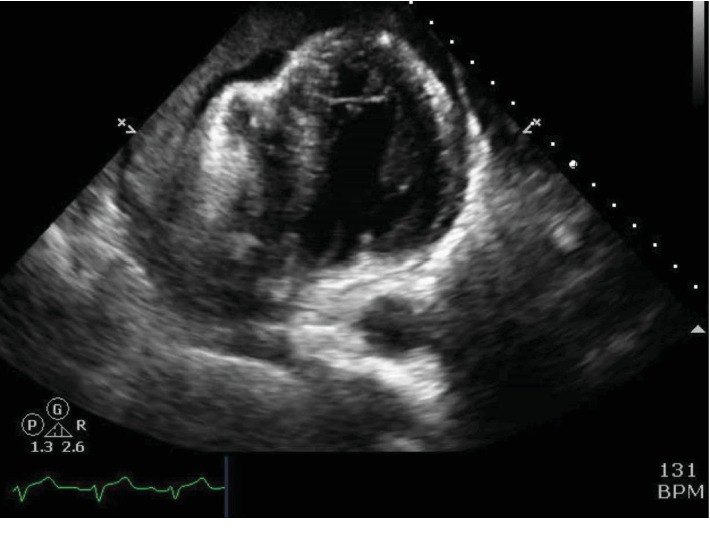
Transesophageal echocardiogram revealing a large pericardial effusion with diastolic compression of the right ventricle.
